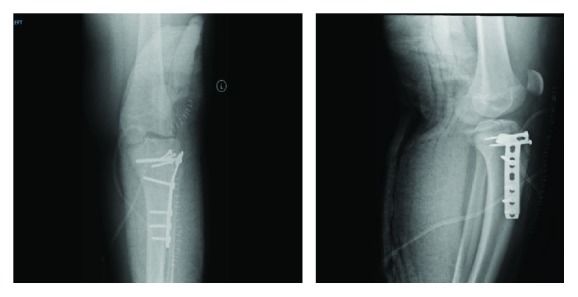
AP and lateral X-ray of the left knee postoperative day 1.
